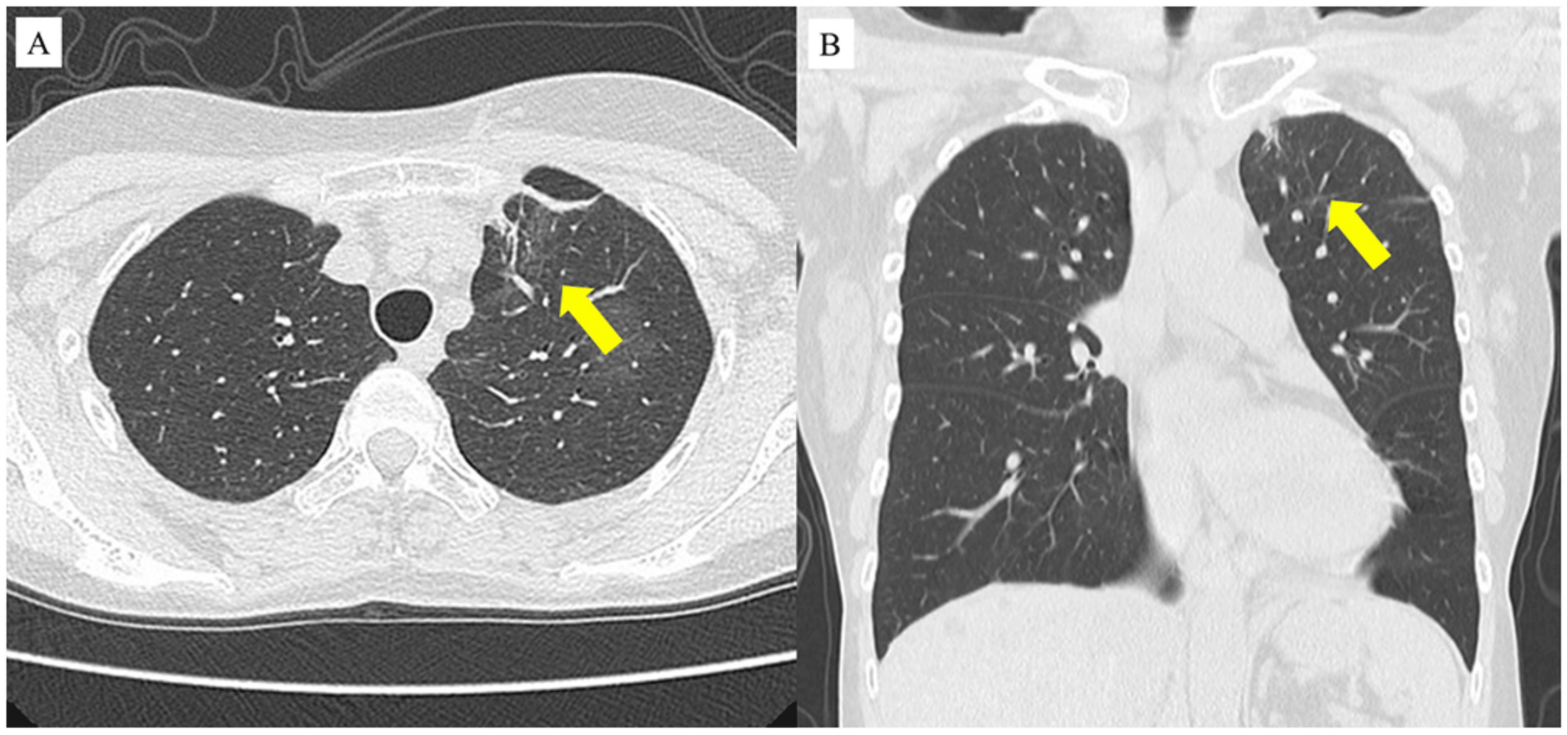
Chest computed tomography after initiating treatment of EGPA and TB EGPA: eosinophilic granulomatosis with polyangiitis; TB: tuberculosis Chest computed tomography shows a reduced area of consolidation and newly emerged scarring shadows eight weeks after initiating treatment for EGPA and pulmonary TB (yellow arrow) (A, B)
